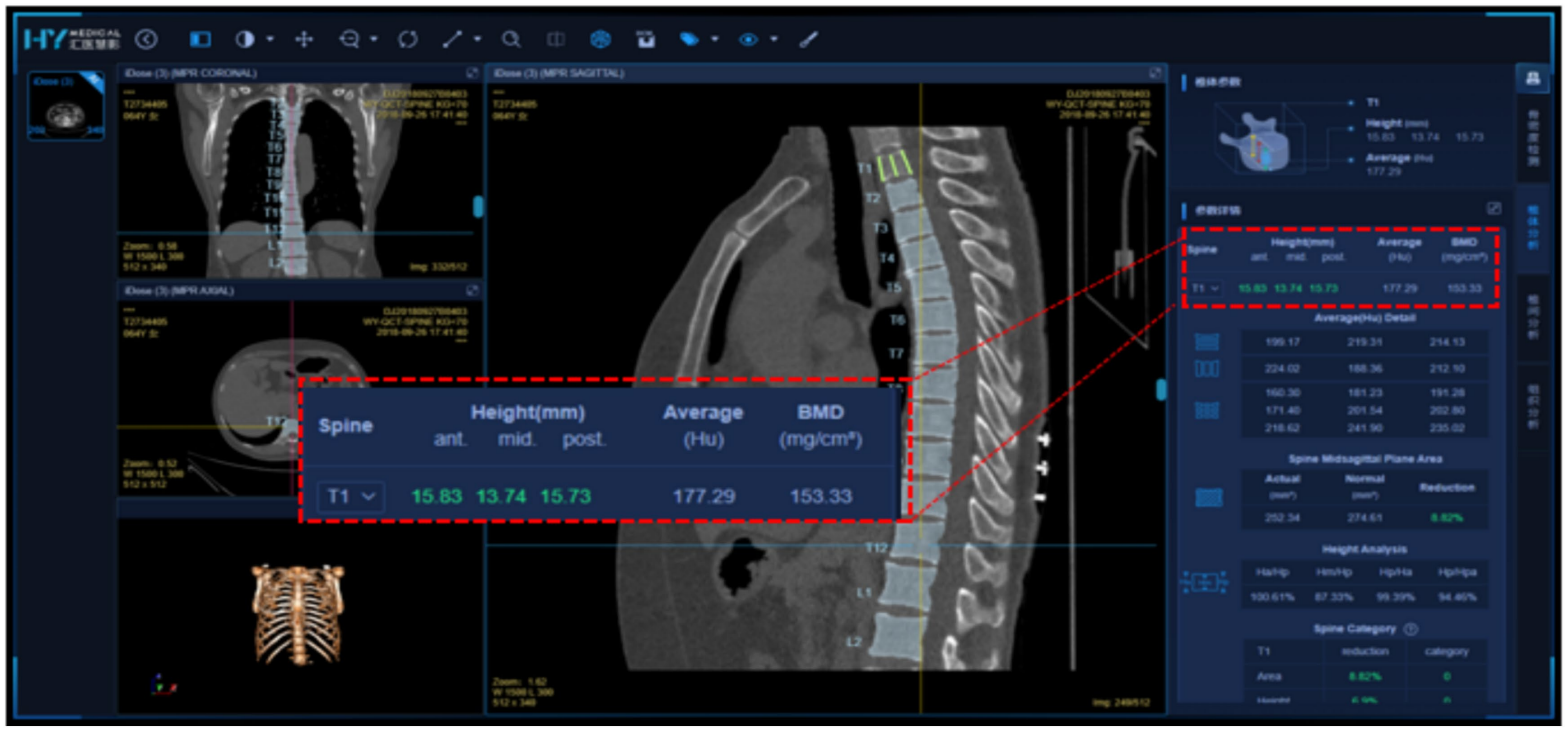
Detailed visualization interface of Huiyi Huiying AI quantitative CT bone density detection software.
